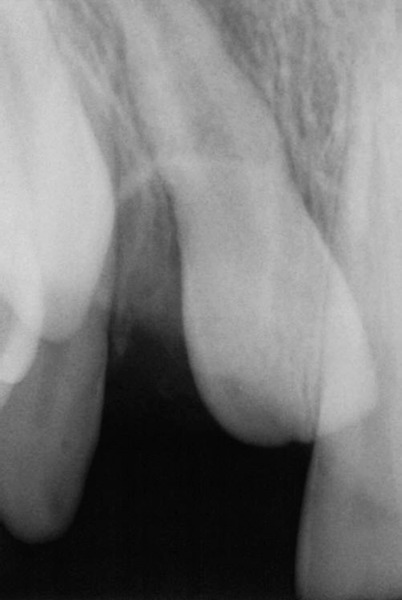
Postoperative radiograph after one week
